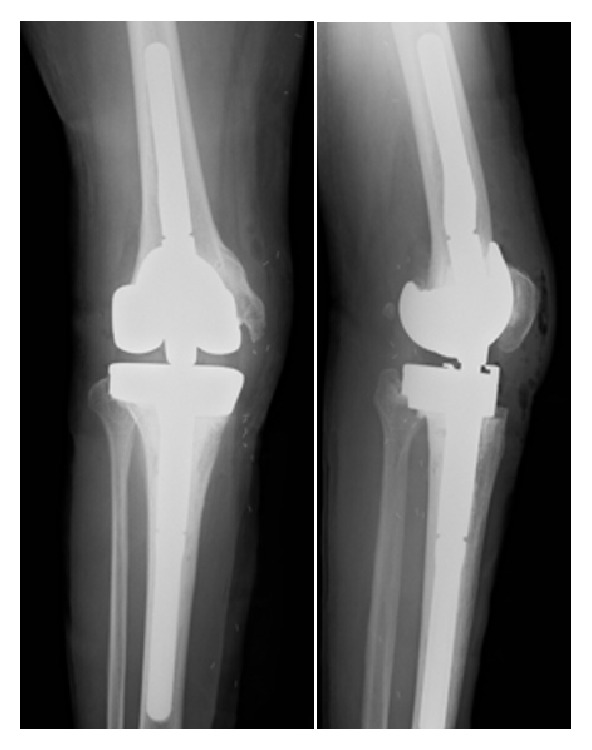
Postoperative anteroposterior radiograph of right knee.
